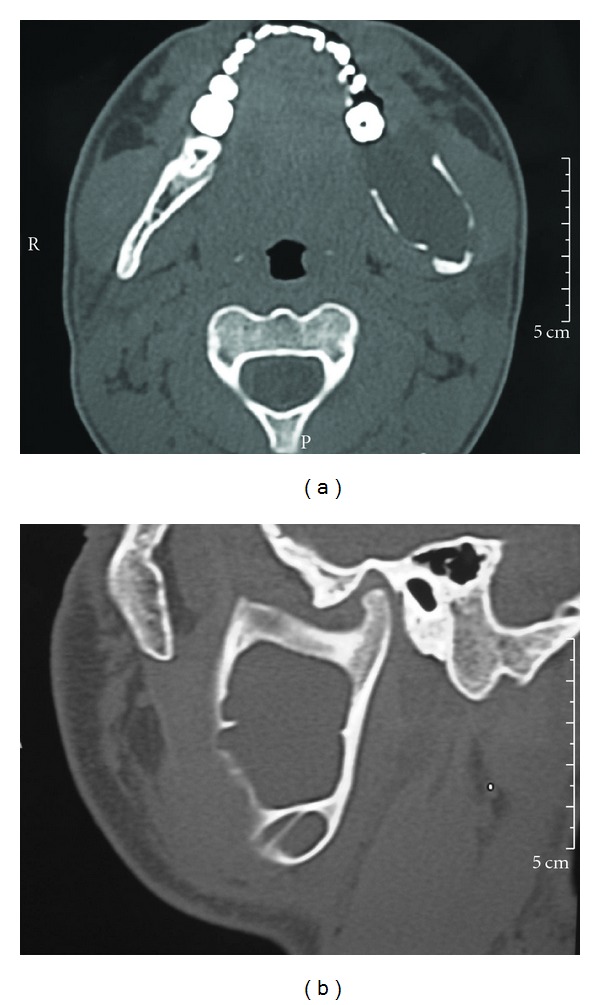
CT axial (a) and sagittal (b) images demonstrate the lingual and buccal cortical expansion and erosion.
